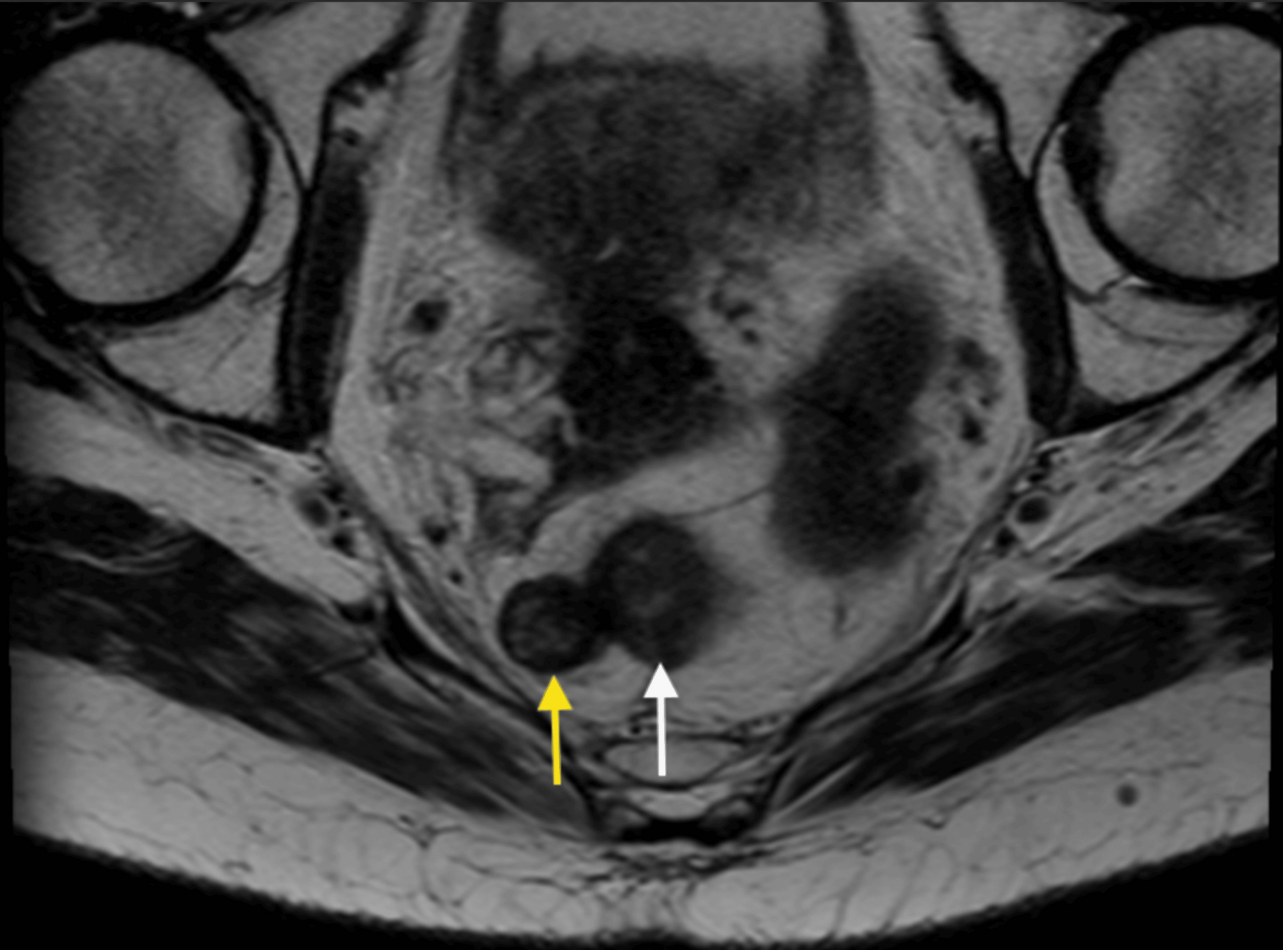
Axial MRI demonstrating a right perirectal T2 heterogeneous mass (yellow arrow) measuring 1.6 x 1.5 cm with T2 hypointense signal and T2 intermediate signal intensity with mild diffusion restriction and heterogeneous enhancement. The mass involves the right lateral rectal wall (white arrow). MRI: magnetic resonance imaging
